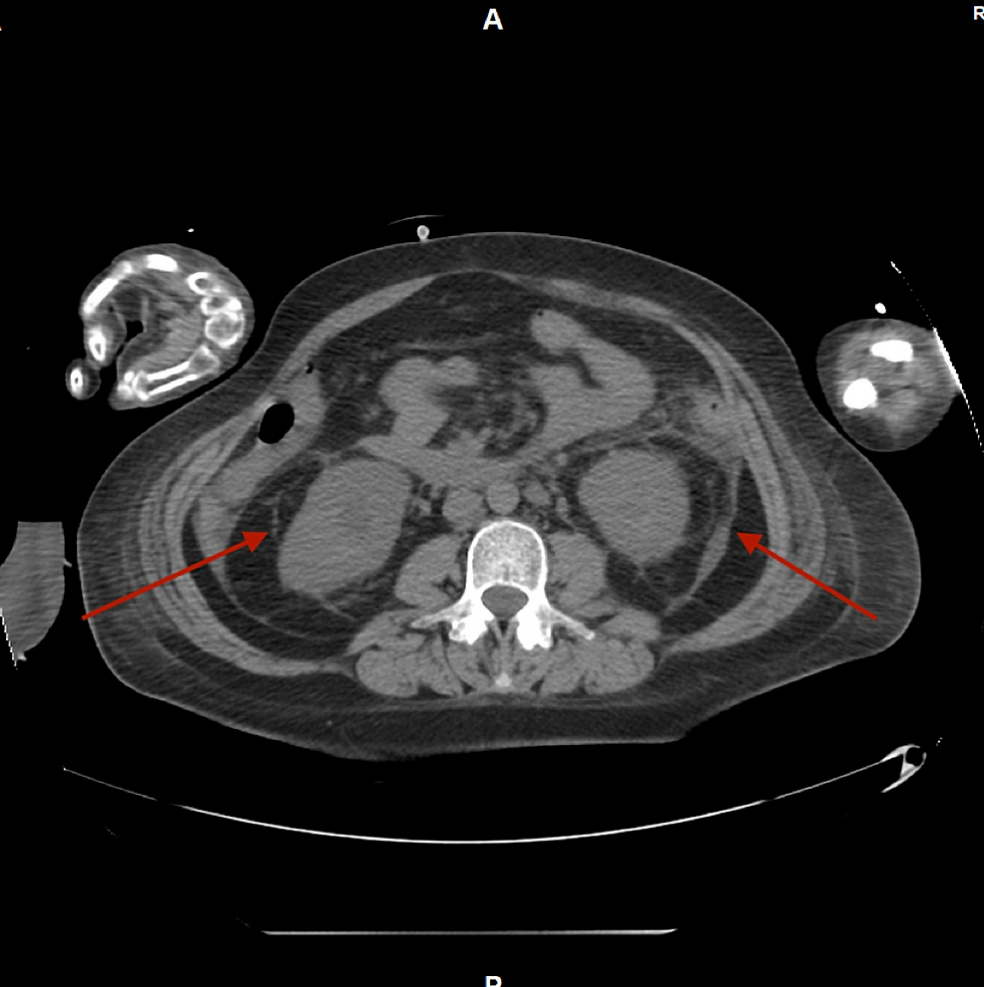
CT scan of the abdomen and pelvis showing bilateral perinephric fat stranding. CT: computed tomography
